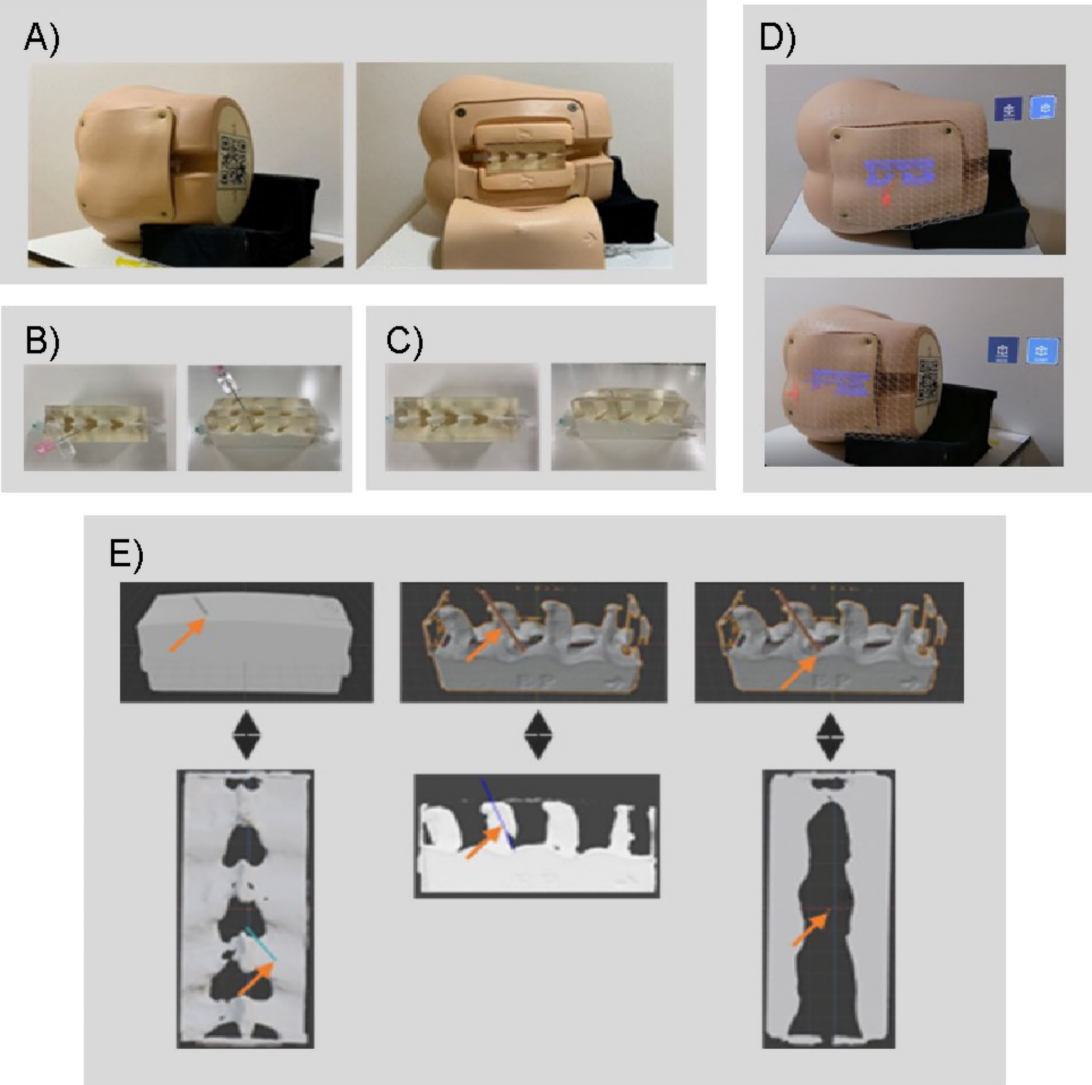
Epidural Anesthesia Practice Kit and ideal Insertion Model and Hologram Visibility. Remove the pad inside the epidural anesthesia practice kit (a). The epidural needle is inserted at the ideal point (b), and the needle is cut with nippers to create the ideal insertion model (c). An AR marker is attached to the head side of the epidural anesthesia practice kit. HoloLens2Ⓡis set to recognize the AR marker, and a hologram of the ideal insertion model is projected at the same position as the pad in the epidural anesthesia practice kit. The red holographic needle shows the ideal epidural needle insertion path (d). The view of the performer using HoloLens2Ⓡ is shown. The 3D model of the surface puncture point, puncture angle, and epidural space puncture point constructed from CT images and cross-sectioned in Blender 2.83.9 (e). Yellow arrows indicate the surface puncture point, puncture angle, and epidural puncture point
